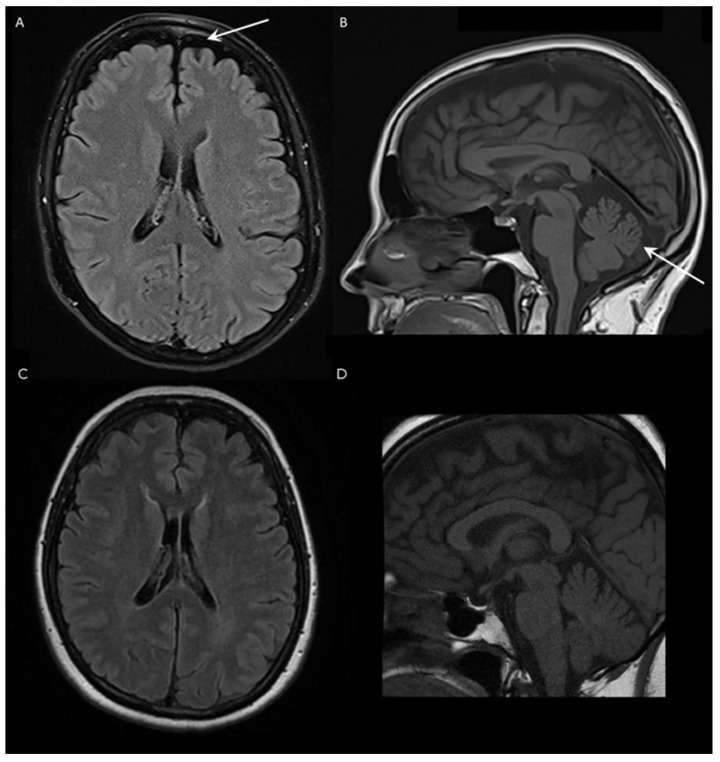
Brain magnetic resonance imaging (MRI) of a Hungarian SCA40 patient and an age-matched healthy subject. (A) Fluid-attenuated inversion recovery (FLAIR) axial and (B) T1-weighted sagittal scans of the patient. The arrows indicate the frontal lobe and cerebellar atrophy, respectively. (C) FLAIR axial and (D) T1-weighted sagittal images of a healthy age-matched female.
